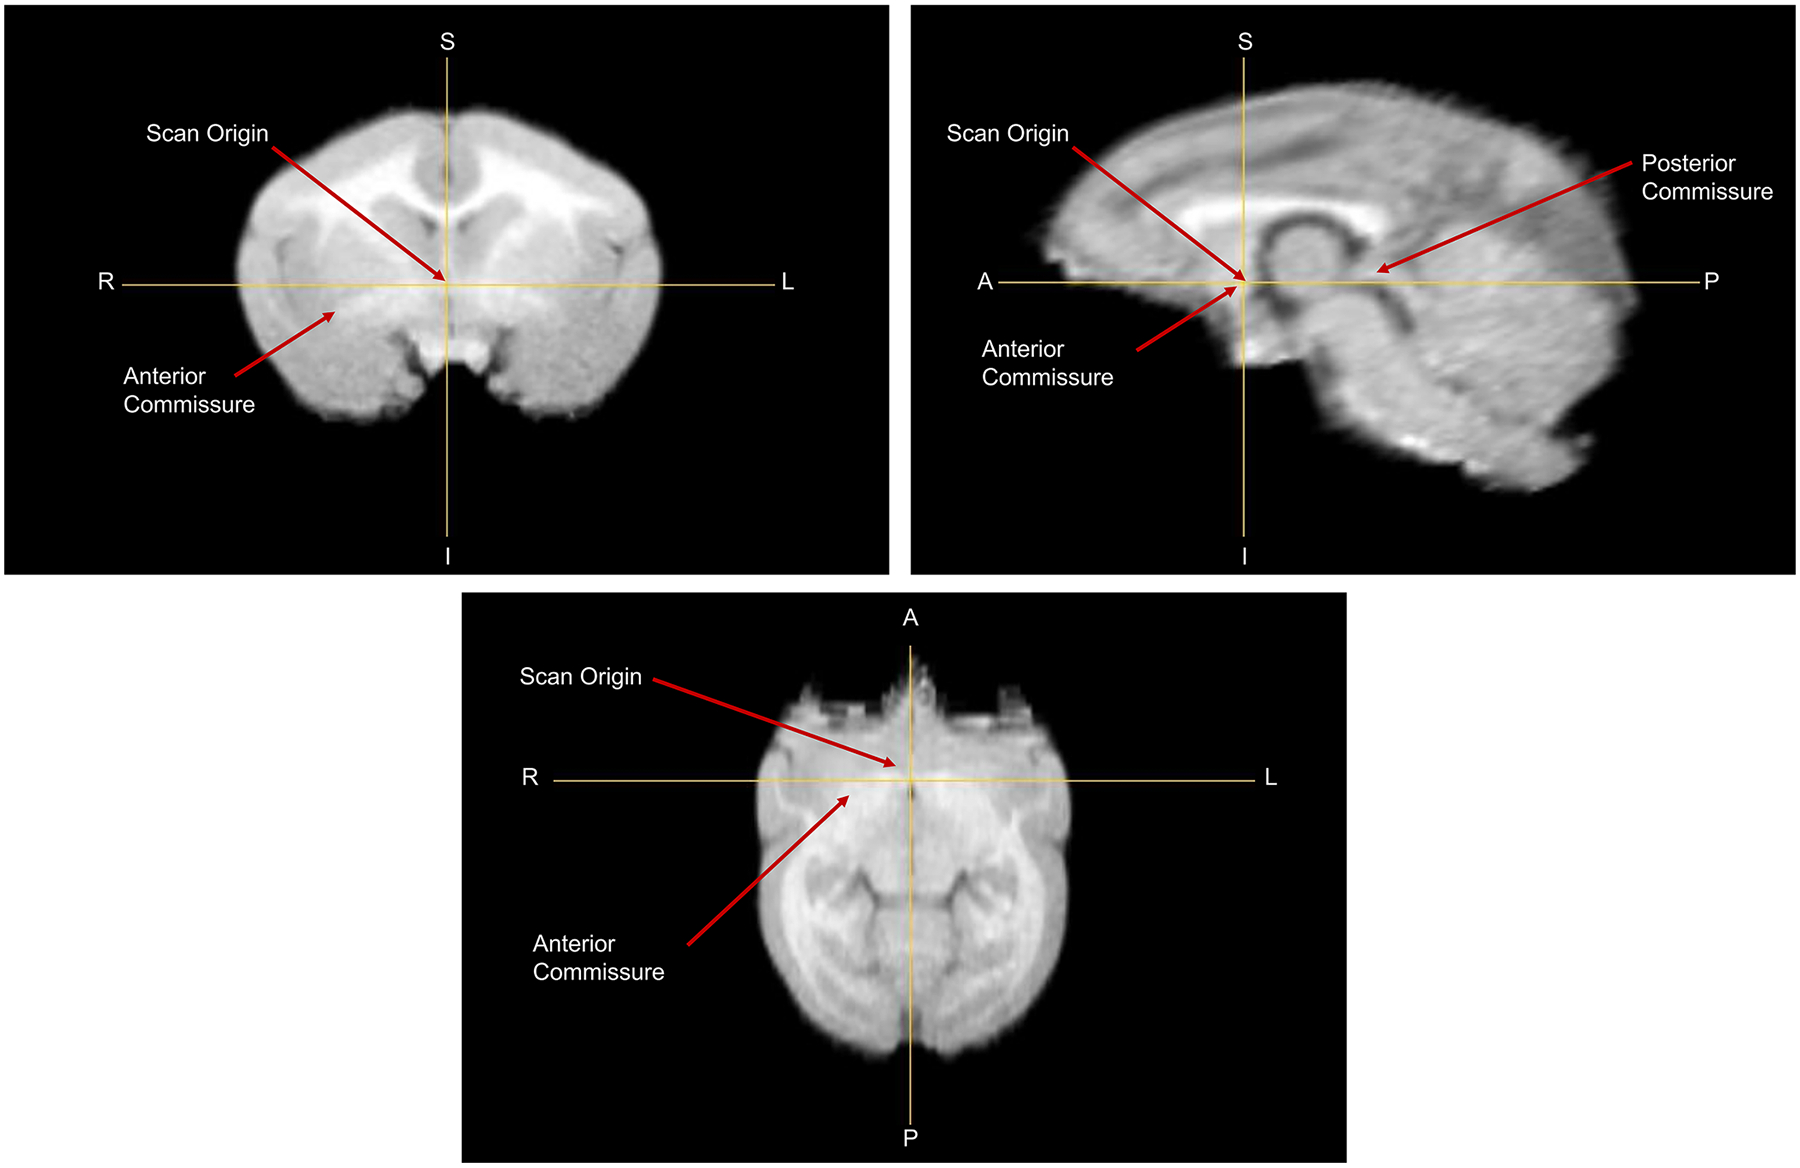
The MRI selected to develop UCD-TiNA was first manually mapped to AC-PC space. The horizontal plane was assigned to be the line connecting the dorsal apex of the anterior commissure to the ventral nadir of the posterior commissure. The apex of the anterior commissure was set to be the origin of the scan.
